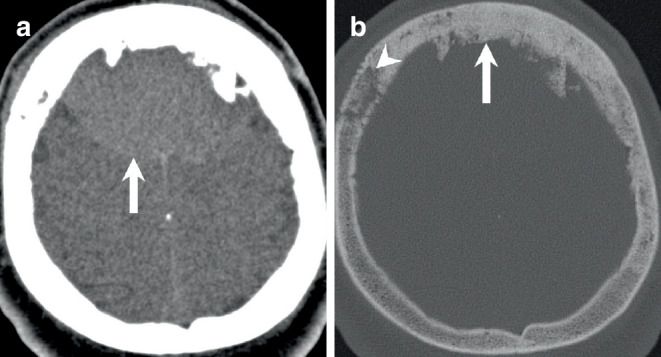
Axial CT images in soft tissue settings (a) obtained upon admission revealed a bifrontal space-occupying lesion, demonstrating slightly higher density compared to brain tissue (arrow). On axial CT images in bone window settings (b), the frontal bone appeared denser and hyperplastic (arrow) with evident osteolytic areas (arrowhead)
